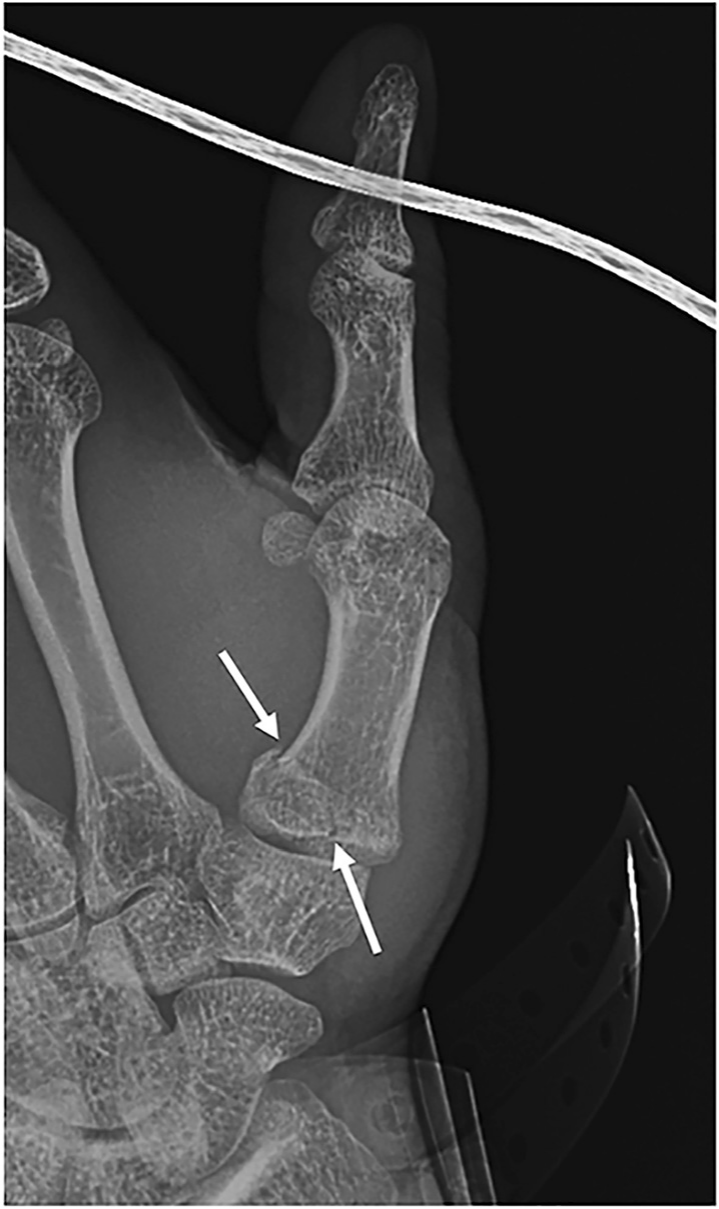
Bennett fracture. This single radiographic view of the thumb demonstrates an oblique intra-articular fracture at the base of the first metacarpal (*arrows*)
